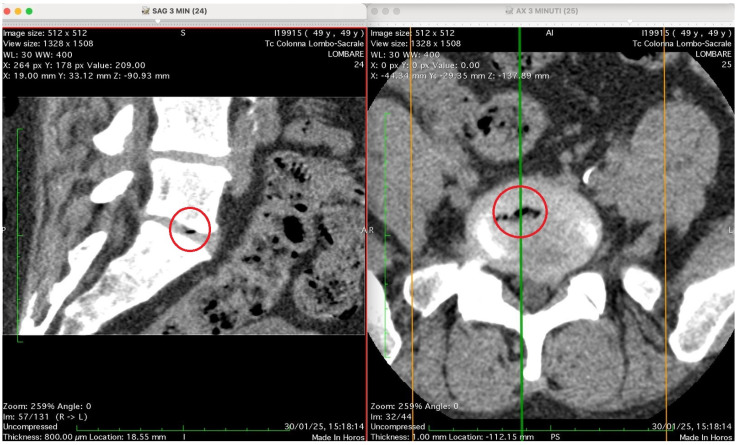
Sagittal (left) and axial (right) CT images of the lumbosacral spine at 3 min post-injection, showing a sharply defined intradiscal gas focus (red circle), likely at the L4–L5 or L5–S1 level. The gas appears as a low-density area within the nucleus pulposus, without adjacent soft-tissue reaction or vertebral endplate disruption. Axial view confirms intradiscal localization with preserved neural structures. Findings are consistent with vacuum phenomenon or gas migration via annular microfissures following paravertebral oxygen–ozone therapy. Technical details: 512 × 512 matrix, 0.8 mm slice thickness, WL/W: 30/400, scan date: 30 January 2025.
